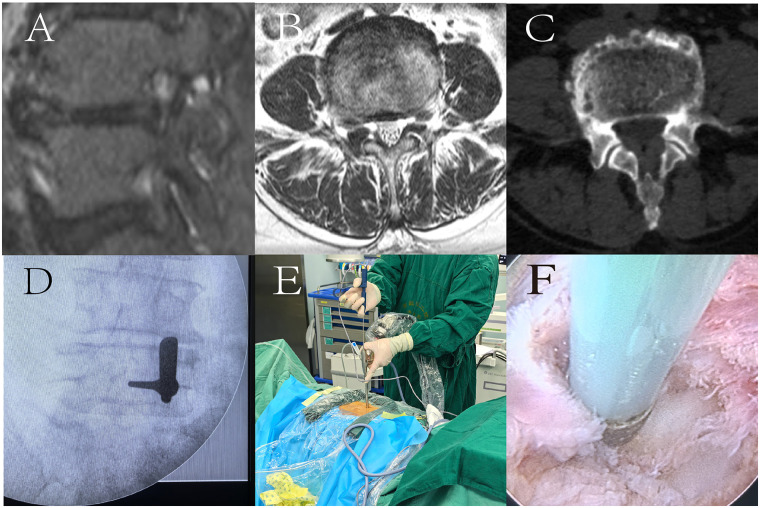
Surgical procedure of RFA and preoperative imaging of patients. (A–C) preoperative MRI and CT showed L4/5 facet joint degeneration. (D–F) D image showed the anteroposterior fluoroscopy utilized during the RFA procedure. Image E showed the RFA working channel. Image F demonstrated the nerve following RFA.
